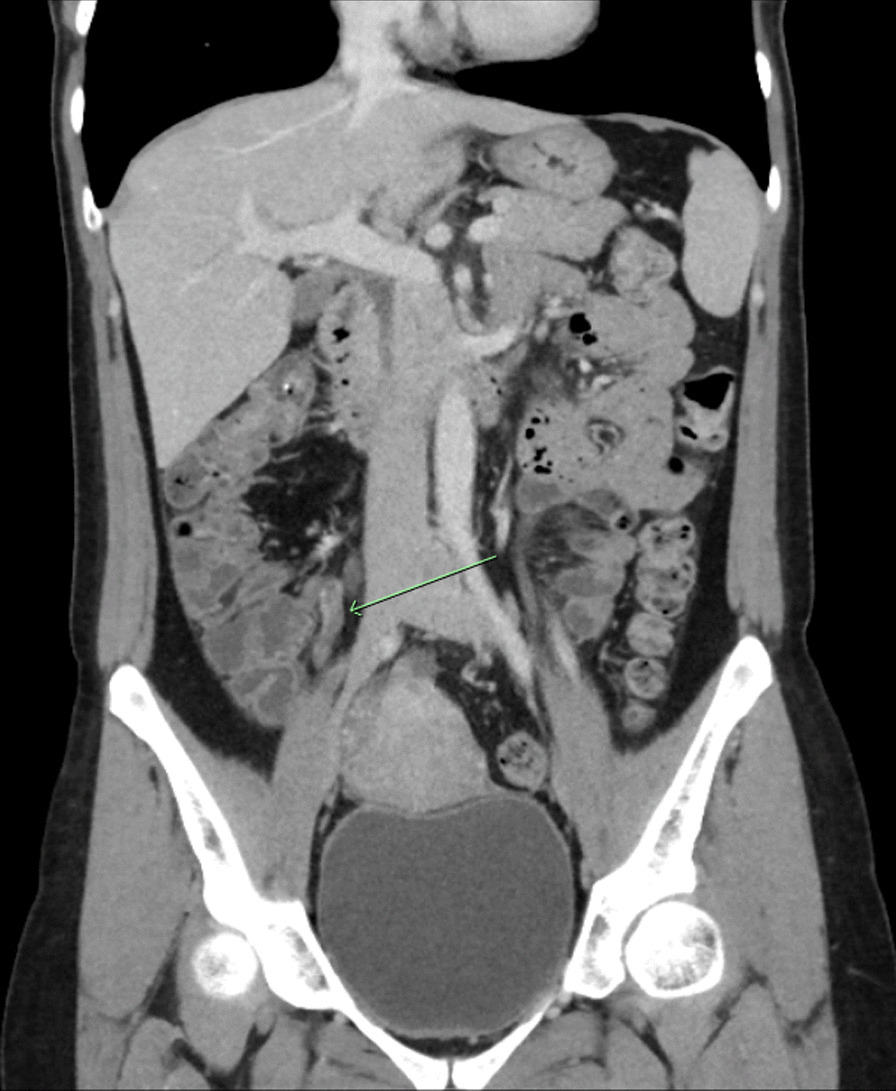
CT abdomen of ovarian vein thrombosis (green arrowing pointing to thrombus)
